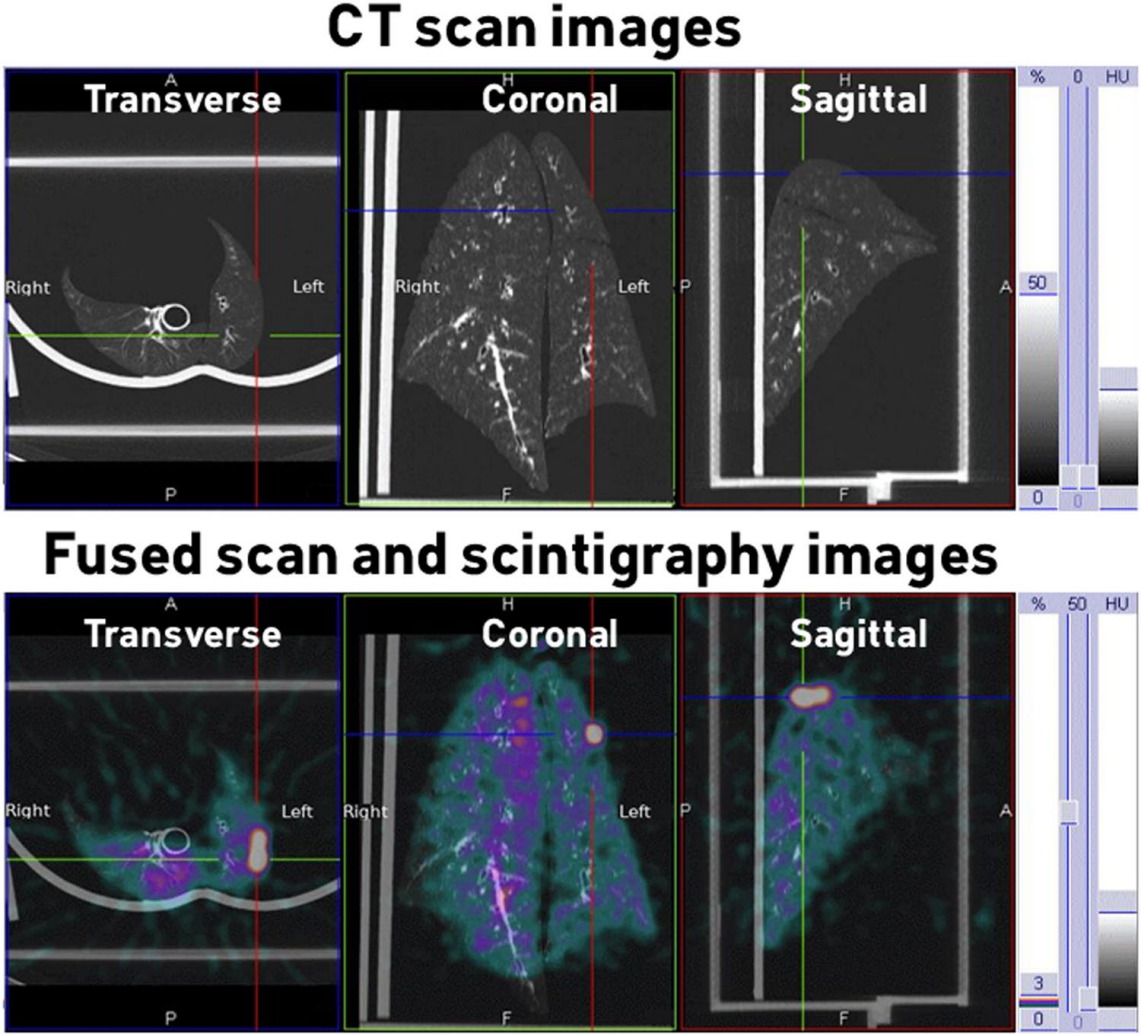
SPECT/CT imaging of one respiratory tract after ^99m^Tc-DTPA nebulization. Upper panels: tomography images. Lower panels: fusion of tomography and scintigraphic images.
